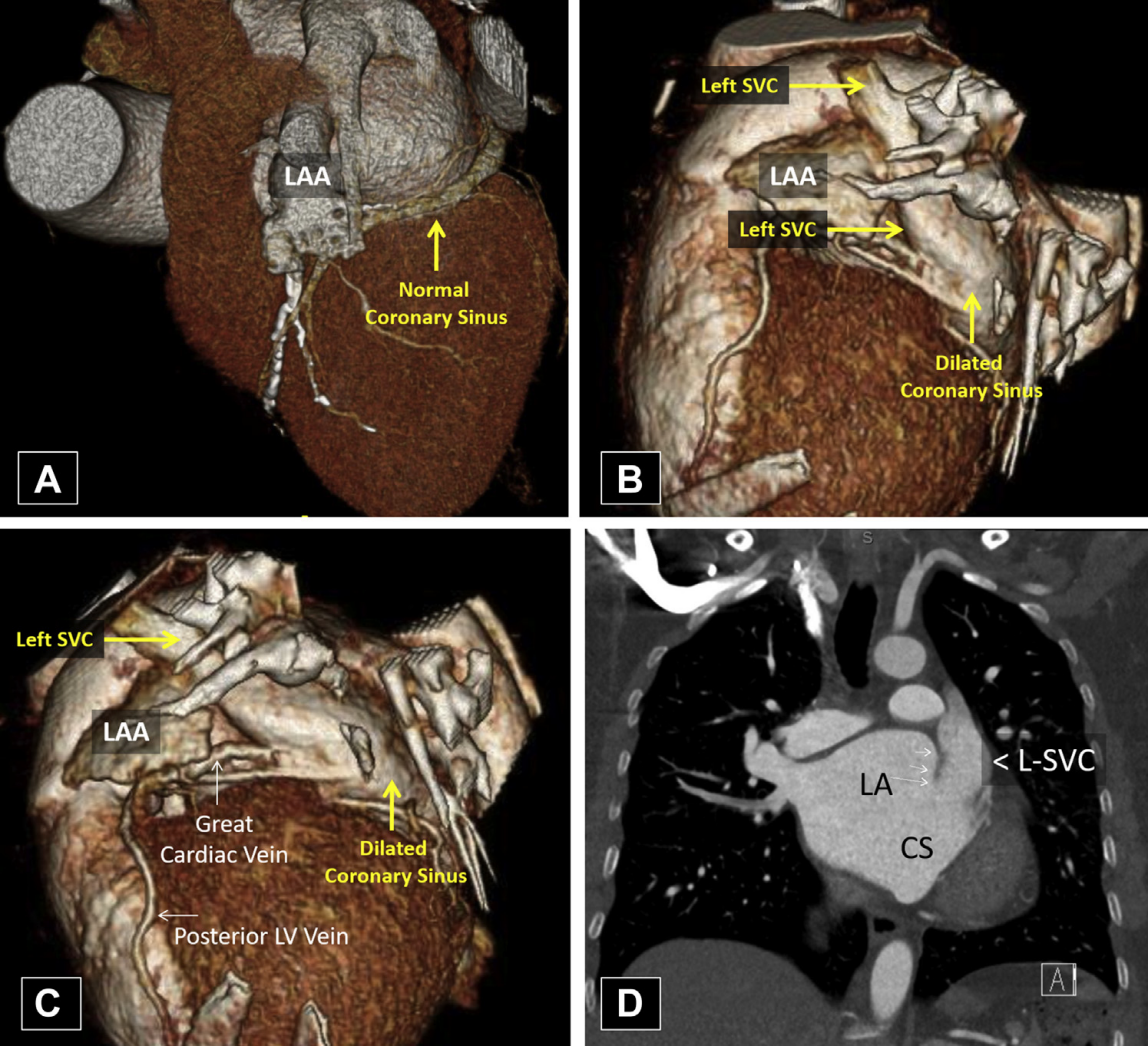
Case 3: persistent L-SVC, unroofed CS. Cardiac CT with three-dimensional reconstruction (A-C) and coronal section (D). This patient's CS (B, C) is markedly dilated when contrasted with a normal CS (A). This is the result of an atypical connection with an L-SVC (B-D) that drains venous blood from the upper body and great cardiac vein via the CS. In addition, this patient's CS appears continuous with the LA (D), indicative of an unroofed CS. This produces a direct connection between the venous system and the left heart and a right-to-left shunt. *CS*, Coronary sinus; *LAA*, left atrial appendage.
